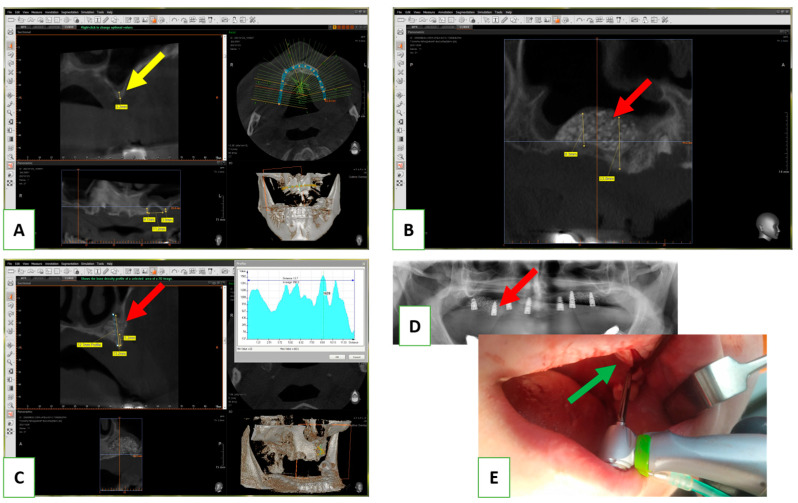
CT scans of a patient with complete edentulism of the upper jaw and a deficiency in bone tissue volume in the lateral sections of the upper jaw before sinus lifting (A); 6 months after the procedure (B,C); and after the dental implant operation (D) with bone sample harvesting (E). Yellow arrow—bone deficiency site; red arrow—HA/β-TCP bioactive nanomaterial; green arrow—place of bone sampling.
